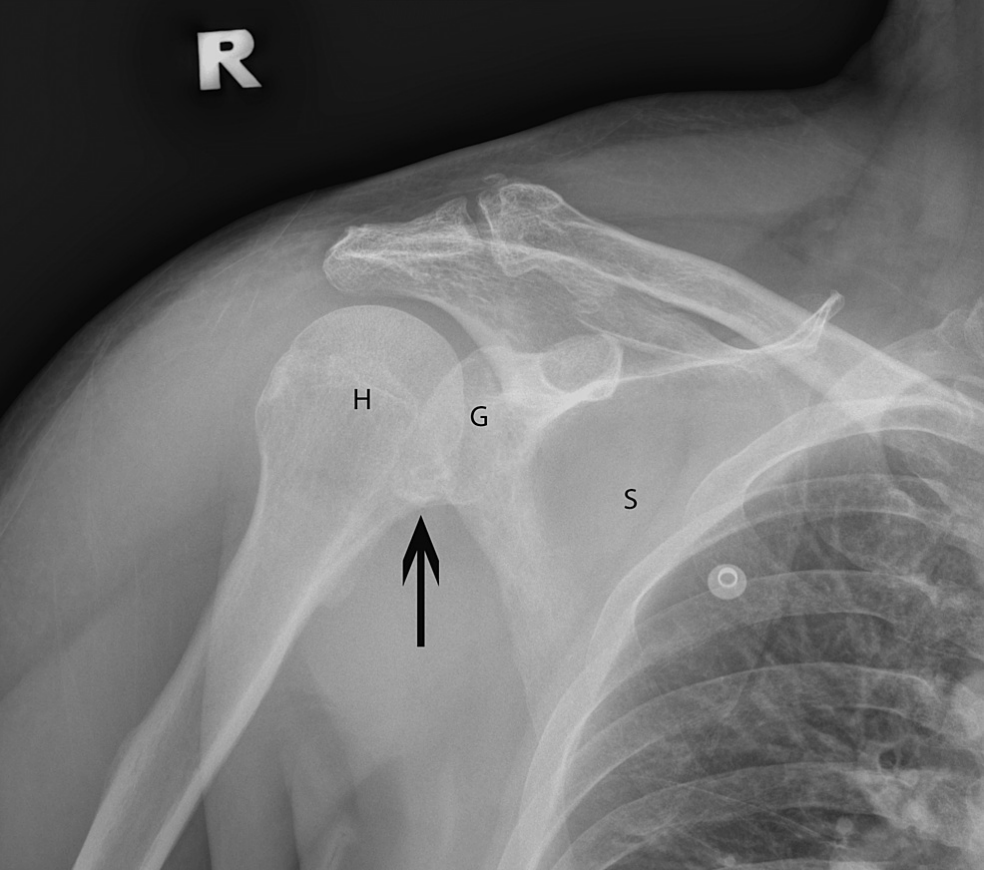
Plain radiograph of the right-shoulder anterior-posterior view demonstrating a Bankart lesion over the glenoid fossa of the right scapula. H: Humerus head; G: Glenoid fossa; S: Scapula; Black arrow: Bankart lesion
